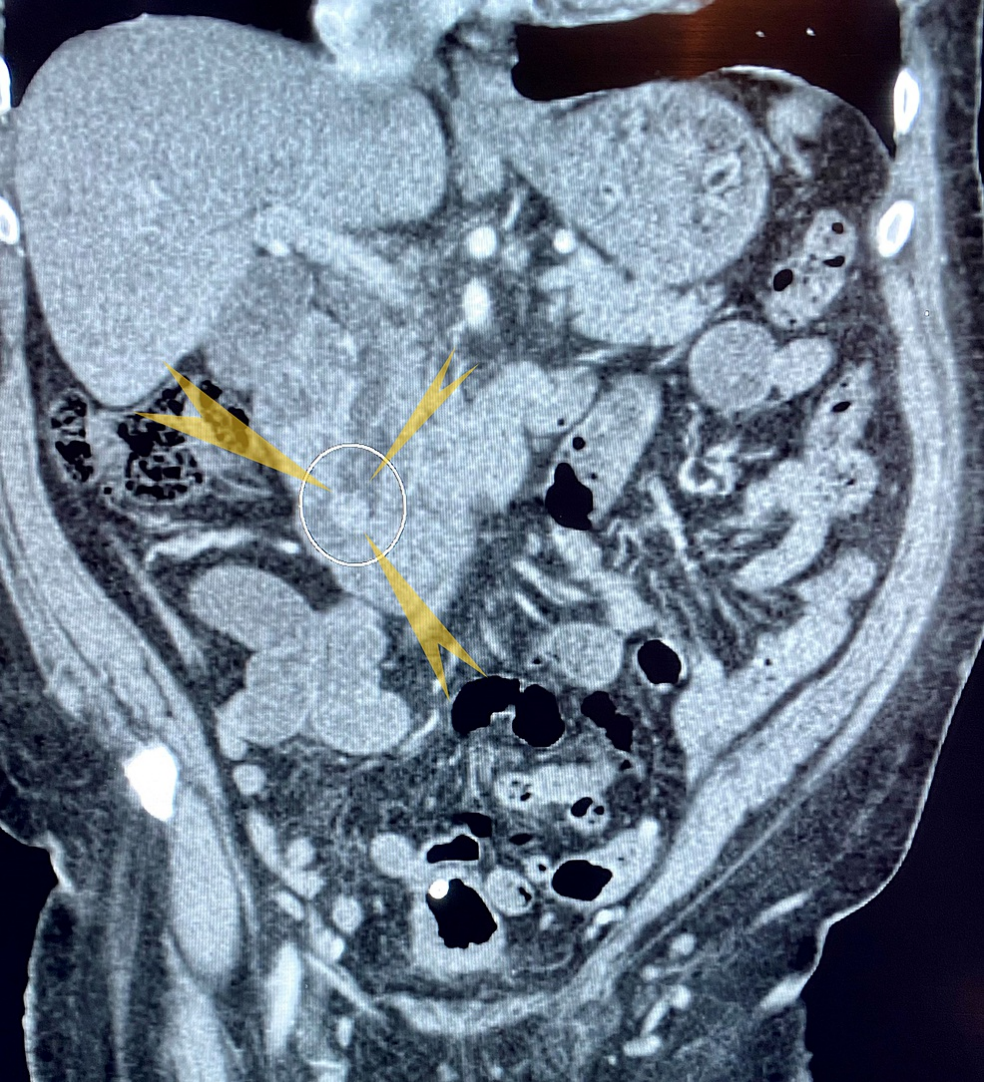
CT abdomen and pelvis with contrast showed a soft tissue density nodule seen at the level of ampulla measuring 1.2 cm X 1.1 cm (yellow arrowheads pointed towards density in the white circle) and common bile duct diameter measured 10 mm and the pancreatic duct was 4.5 mm at the level of the pancreatic head.
